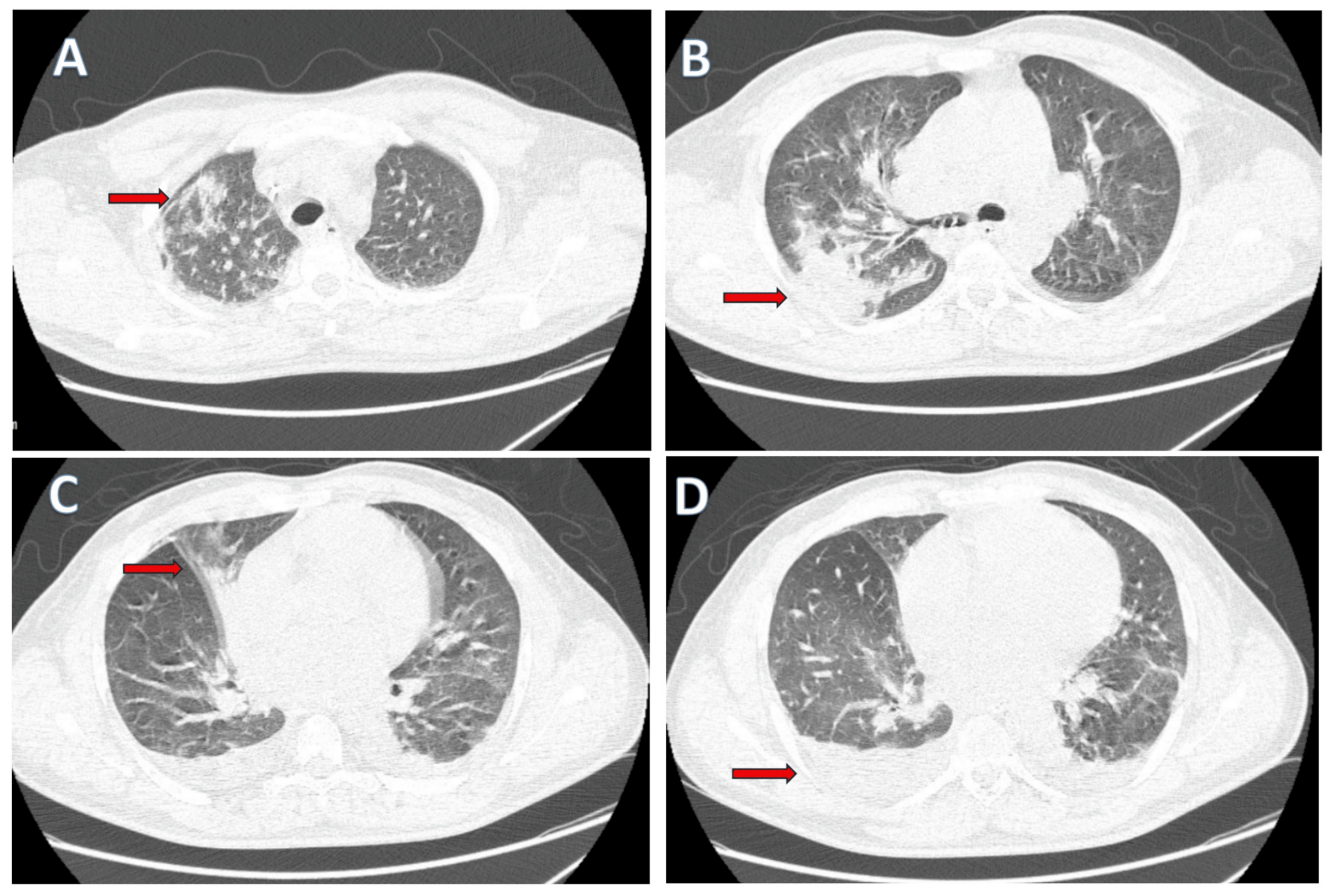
HRCT of the chest showing bilateral pulmonary involvement in a patient with severe scrub typhus pneumonia. (A) Axial HRCT at upper lung zone demonstrating bilateral patchy alveolar opacities, predominantly in the right upper lobe (red arrow). (B) Middle lung zone showing extensive bilateral ground-glass opacities and consolidation, more prominent in the right middle lobe (red arrow). (C) HRCT image at mid-lung fields reveals segmental consolidation with peribronchial thickening and interstitial infiltrates in the right lung (red arrow), indicating progression of pneumonitis. (D) Lower lung zones showing bilateral mild pleural effusion (red arrow). HRCT: high-resolution computed tomography
